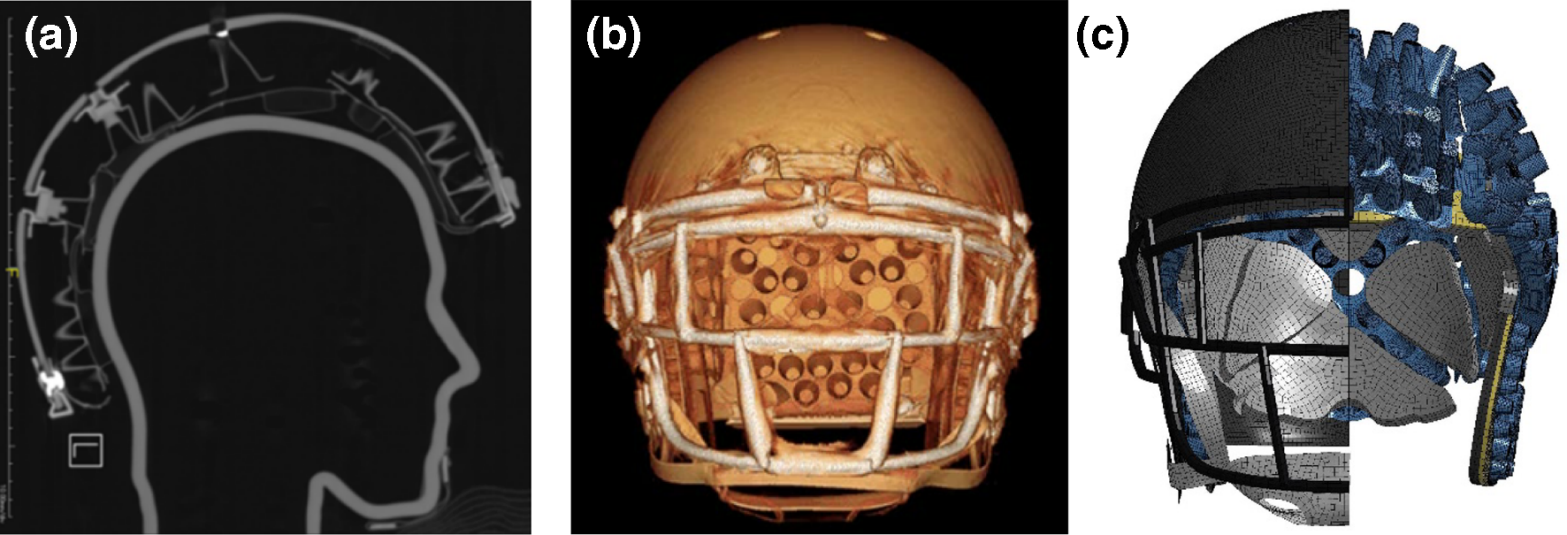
(a) Sagittal cross-section of a CT scan donned on a custom 3D printed NOCSAE head form replica; (b) rendered view of the CT scan of the full helmet and (c) split view of the different components of the helmet mesh.
